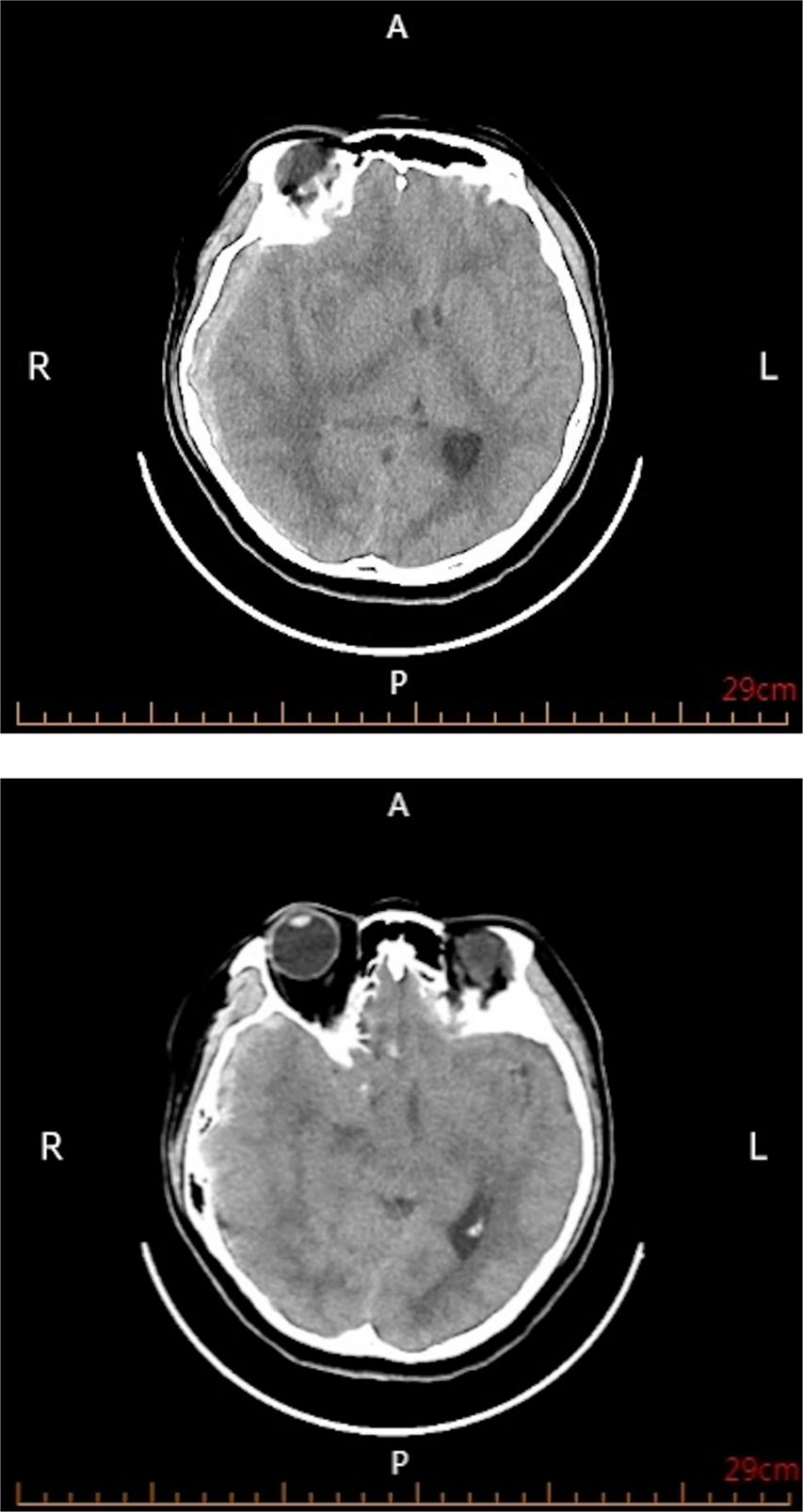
Preoperative non-contrast head CT. R, right; L, left; A, anterior; P, posterior.
